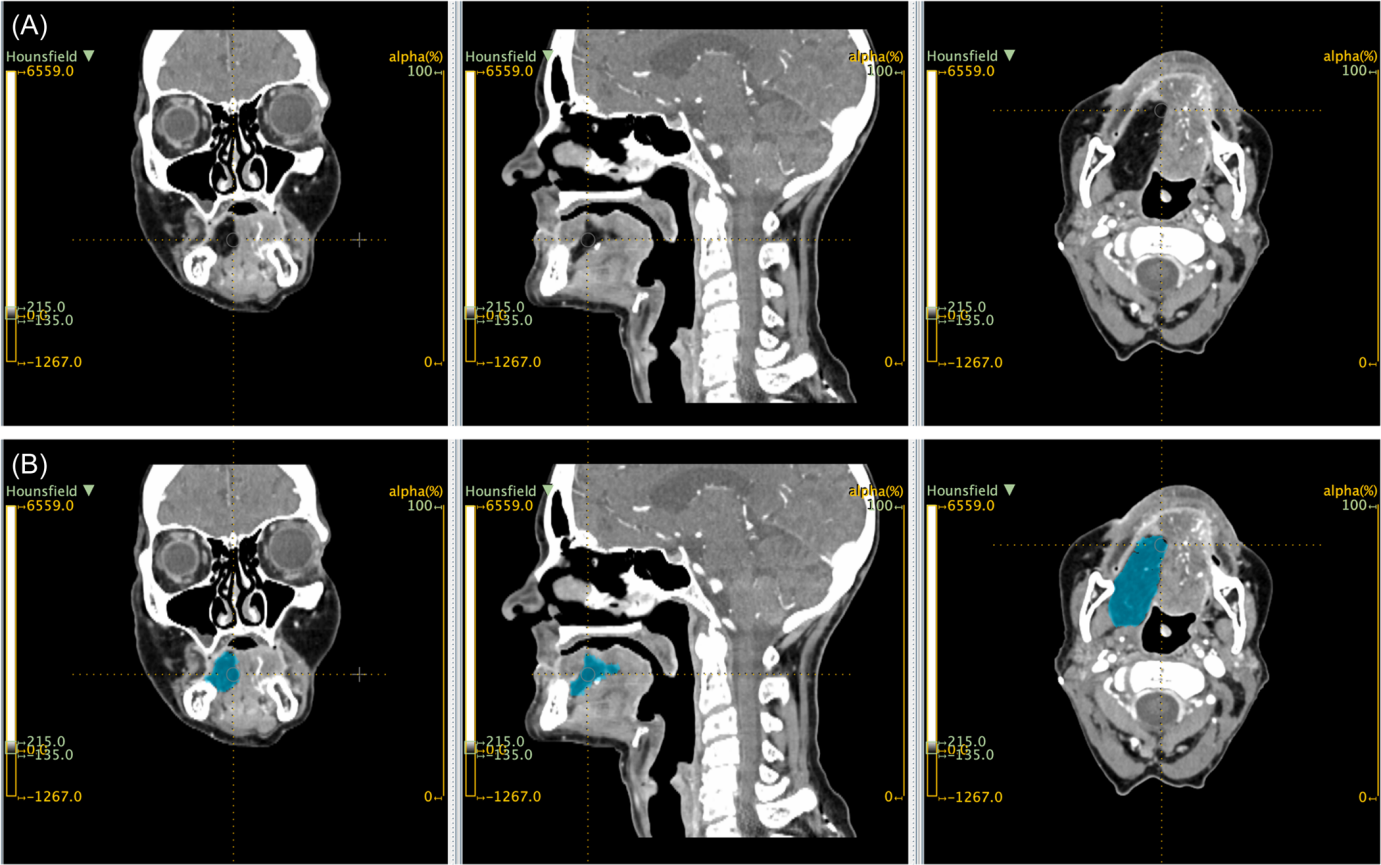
Representative CT imaging imported into LIFEx showing planes of coronal, sagittal and axial views (A) before texture analysis, and (B) after 3D ROI generation of free flap fat volume. 3D, 3‐dimensional; CT, computed tomography; ROI, regions of interest.
